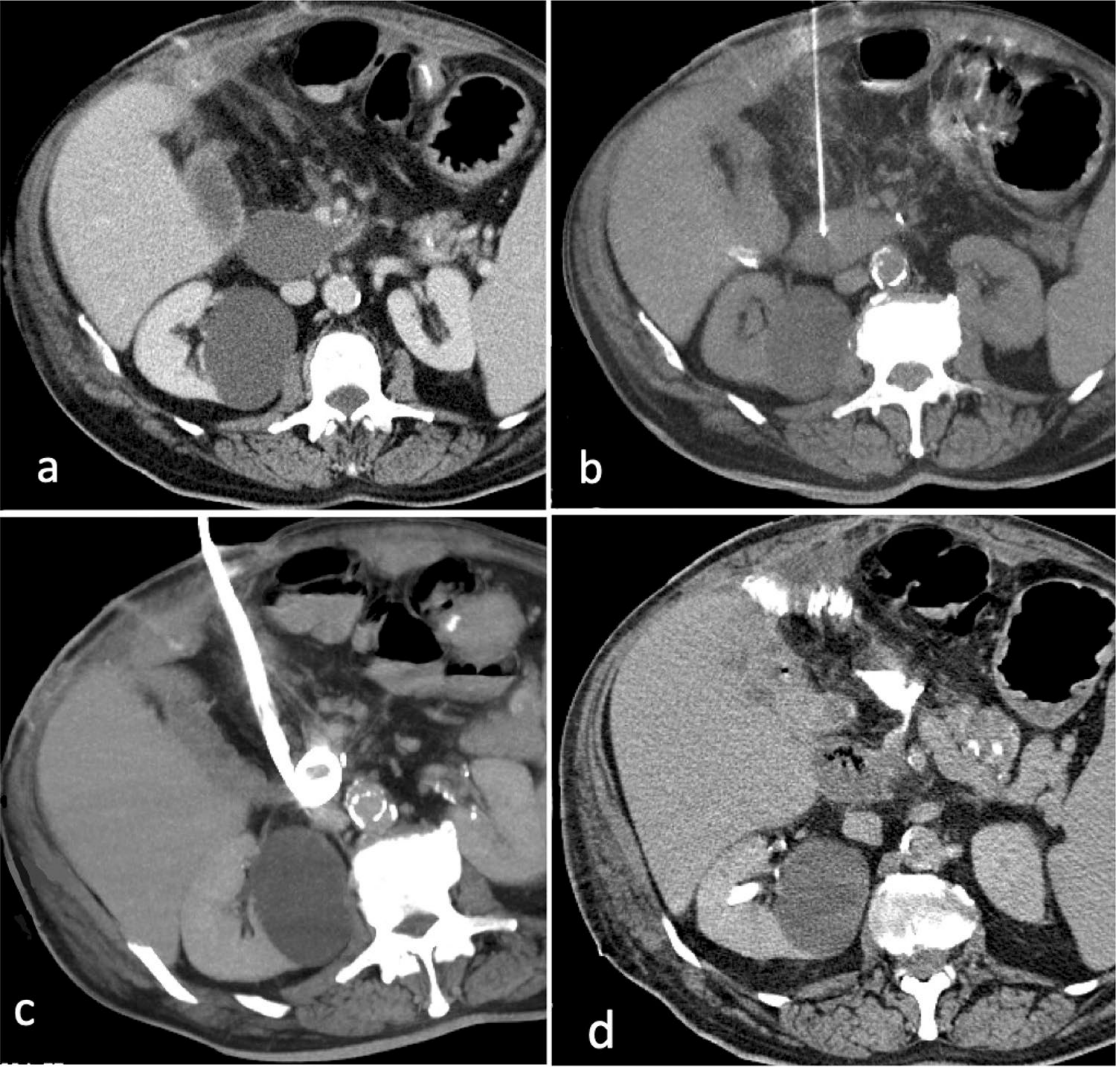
67-year-old male patient with a fluid collection in the inferior duodenal fossa, in proximity of the inferior vena cava, aorta and mesenteric vessels, after pancreatic surgery (a). After the puncture with a chiba needle (b), a 12F PIGTAIL catheter is placed (c: MIP reconstruction) using the Seldinger technique. Control CT scan after 2 weeks shows complete resolution of the fluid collection (d)
